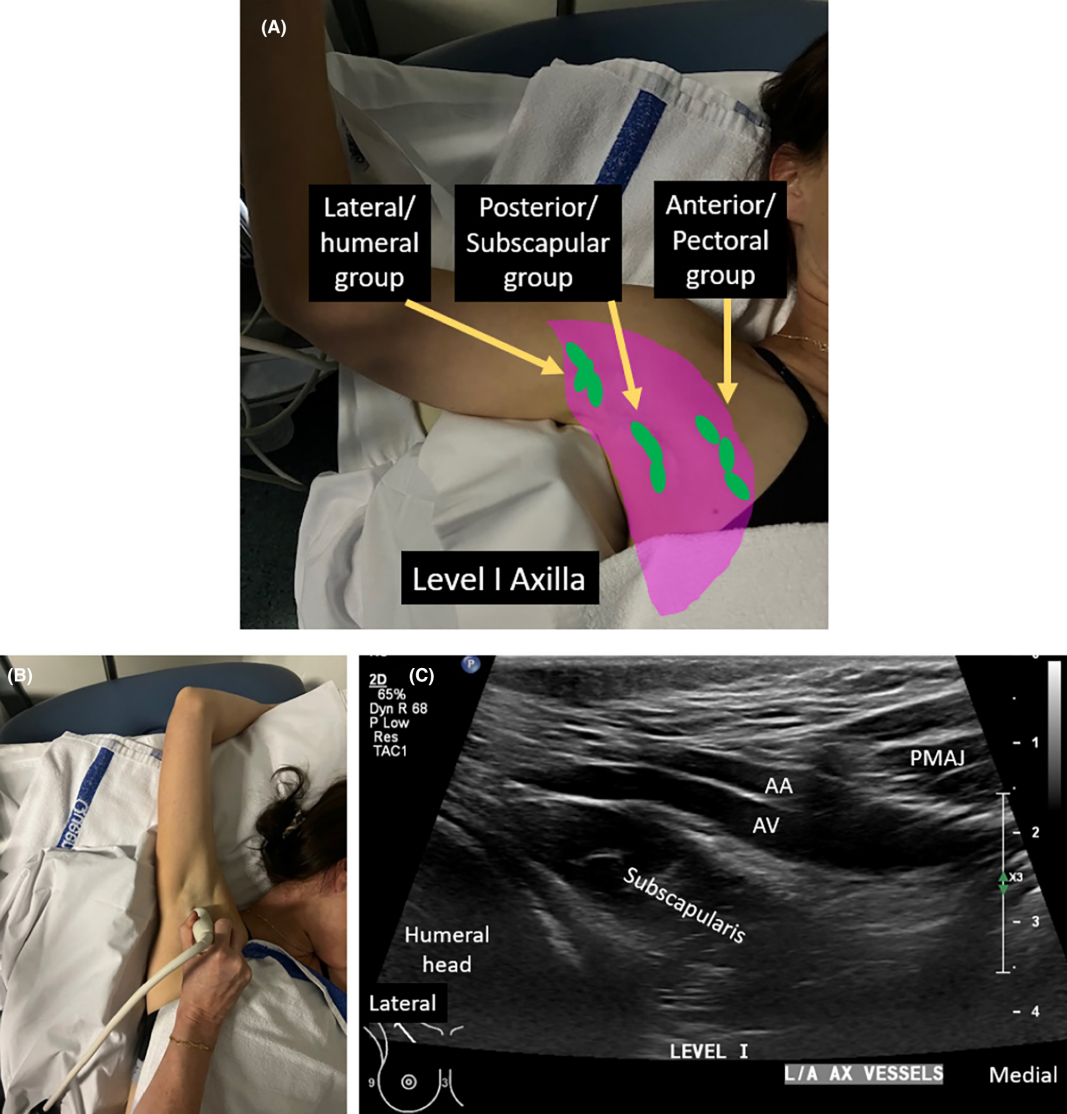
Level I of the axilla. (A). Level I of the axilla outlined by pink. Three groups of lymph nodes are contained within this level which include the anterior/pectoral group, posterior/subscapular group, and the lateral/humeral group. (B). Position of arm and transducer for long‐axis imaging. (C). Sonographic image of Level I the axilla oriented along the long axis of the axillary vessels. AA, axillary artery; AV, axillary vein; PMAJ, pectoralis major muscle.
